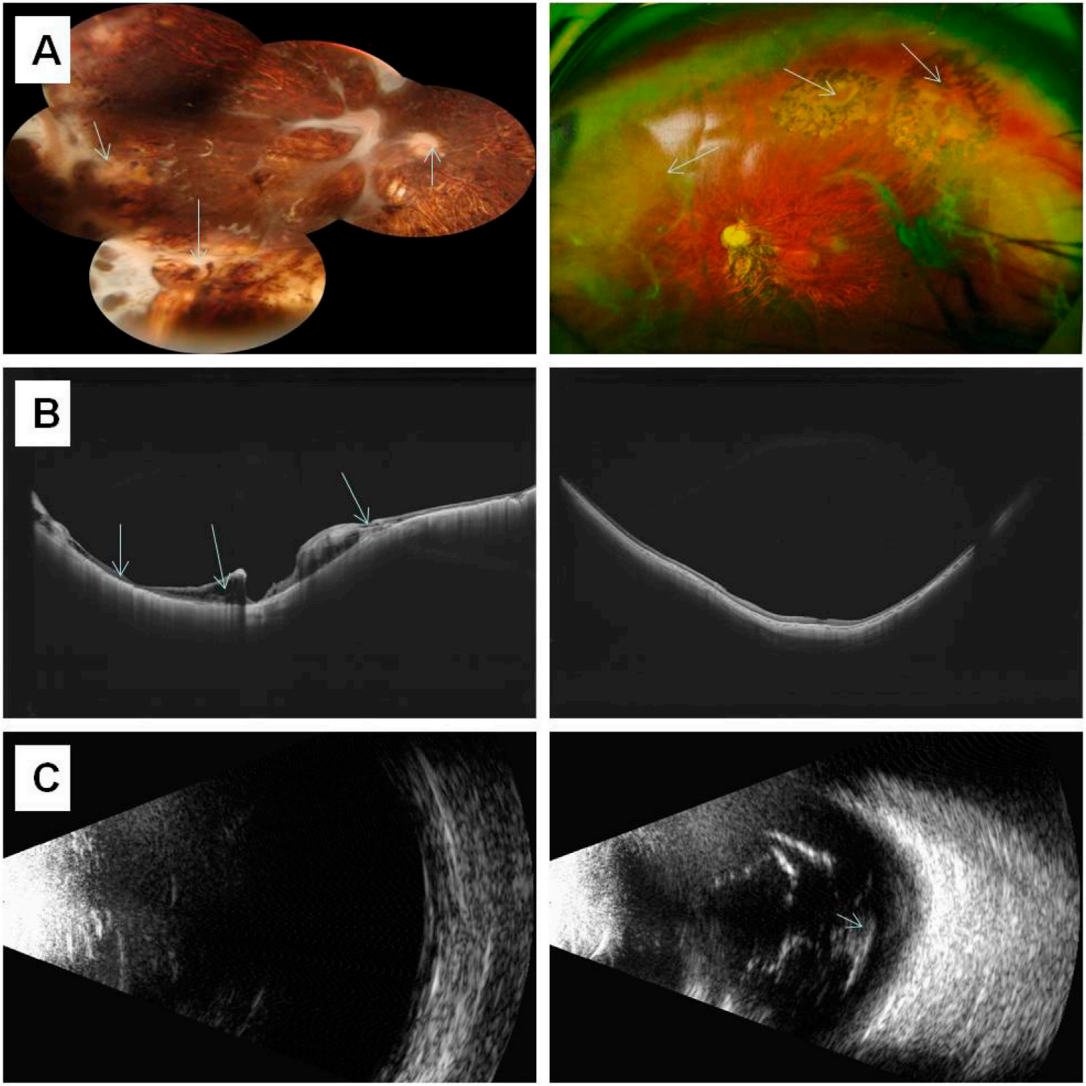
The results of ocular imaging examination of individual I-2 (A) The left and right sides represent the fundus images of the right eye and left eye, respectively; (B) The left and right sides indicate the OCT of the right eye and left eye, respectively; (C) The left and right sides indicate the ocular B-ultrasound examination of the right eye and left eye, respectively. The arrow indicates the lesion.
